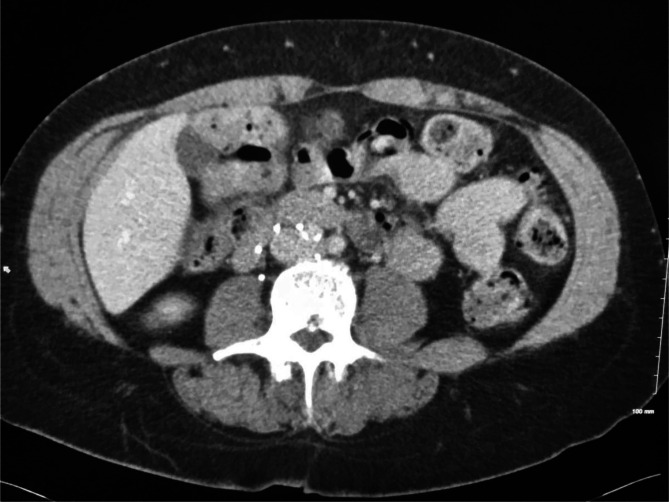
Axial CT demonstrates IVC filter wall penetration of an anchoring strut
